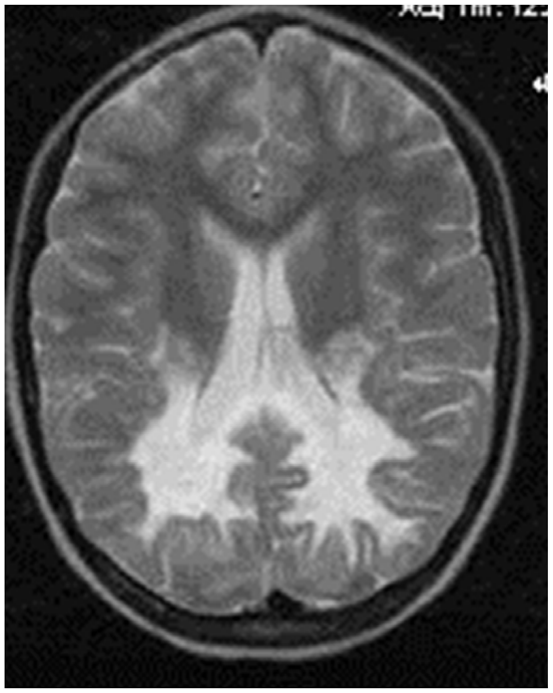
MRI of the female patient 54, showing the typical pattern of white matter abnormality found in X-ALD.
